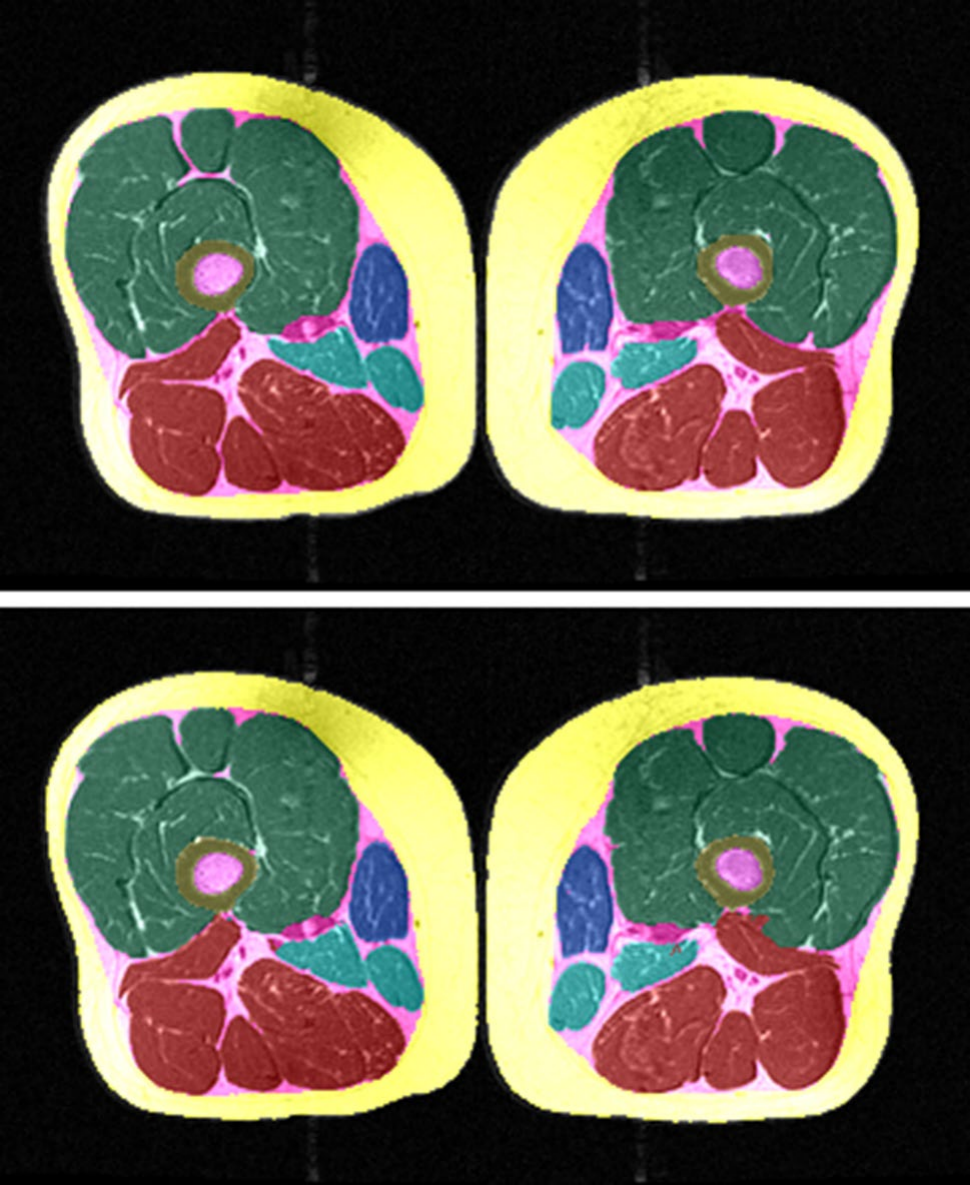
Side differences using manual und U-Net segmentation techniques of thigh MRI (33% distal–proximal) in bilateral knees with the same radiographic disease stage, but unilateral frequent pain; painful knee (right side); painless knee (left side); manual segmentation (upper) and U-Net segmentation (lower)
